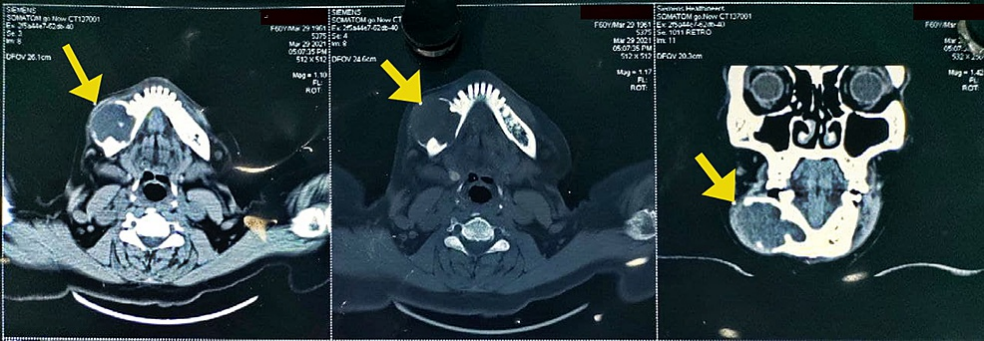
CT scan sections showing well-defined encapsulated homogeneously enhancing lesions in the right body of the mandible.
